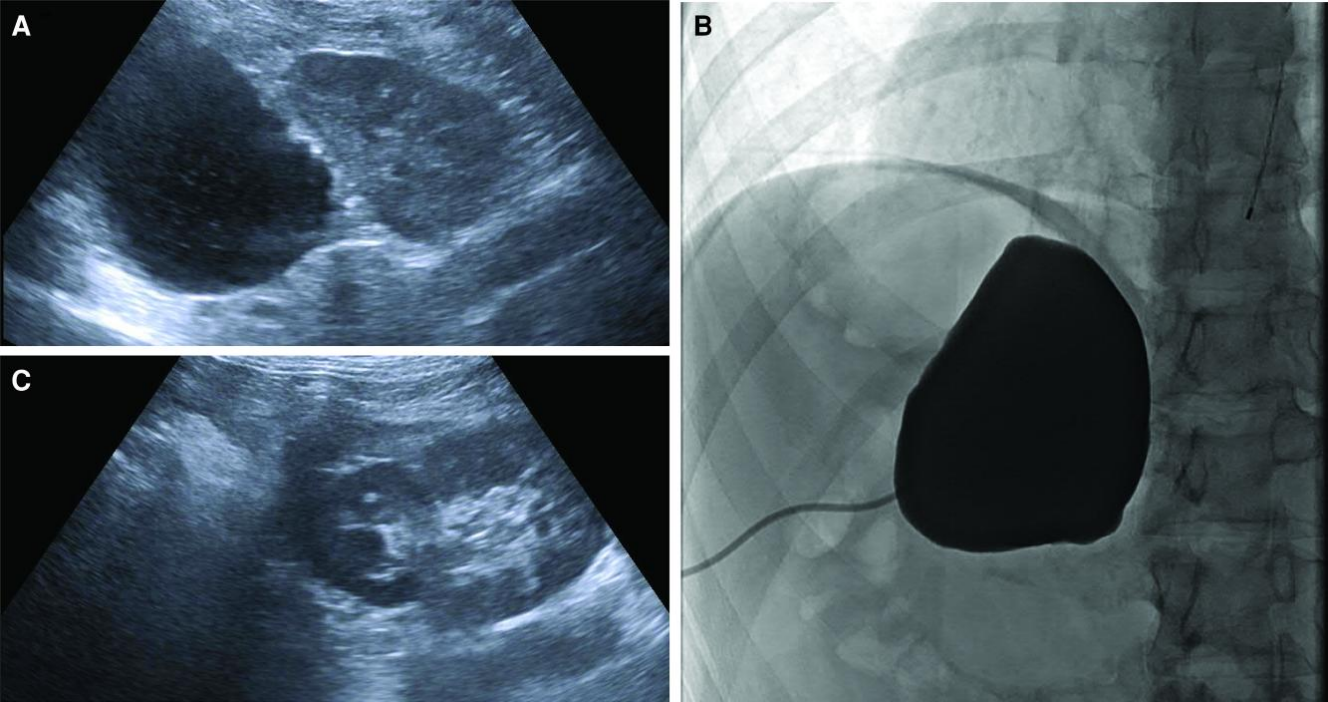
Cyst aspiration and sclerotherapy. (A) Left flank ultrasound demonstrating unilocular anechoic left adrenal cyst. (B) Fluoroscopic images following cyst distention with iodinated contrast. (C) Postaspiration and sclerotherapy ultrasound of left flank demonstrating complete cyst collapse.
